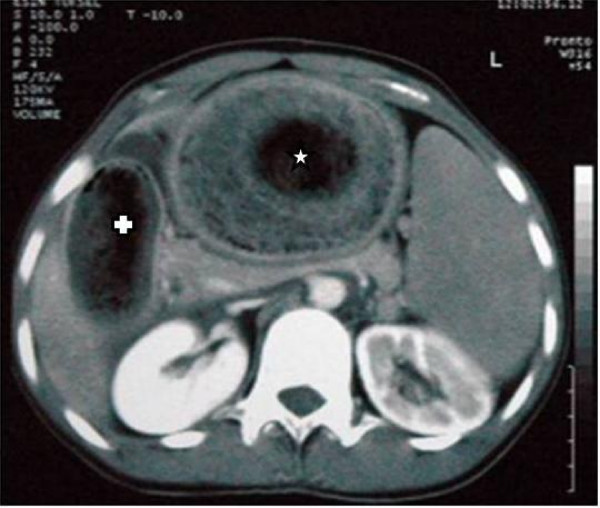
Computed tomogram of the Abdomen. Trichobezoar seen on Stomach (☆) and duodenum (✞)
